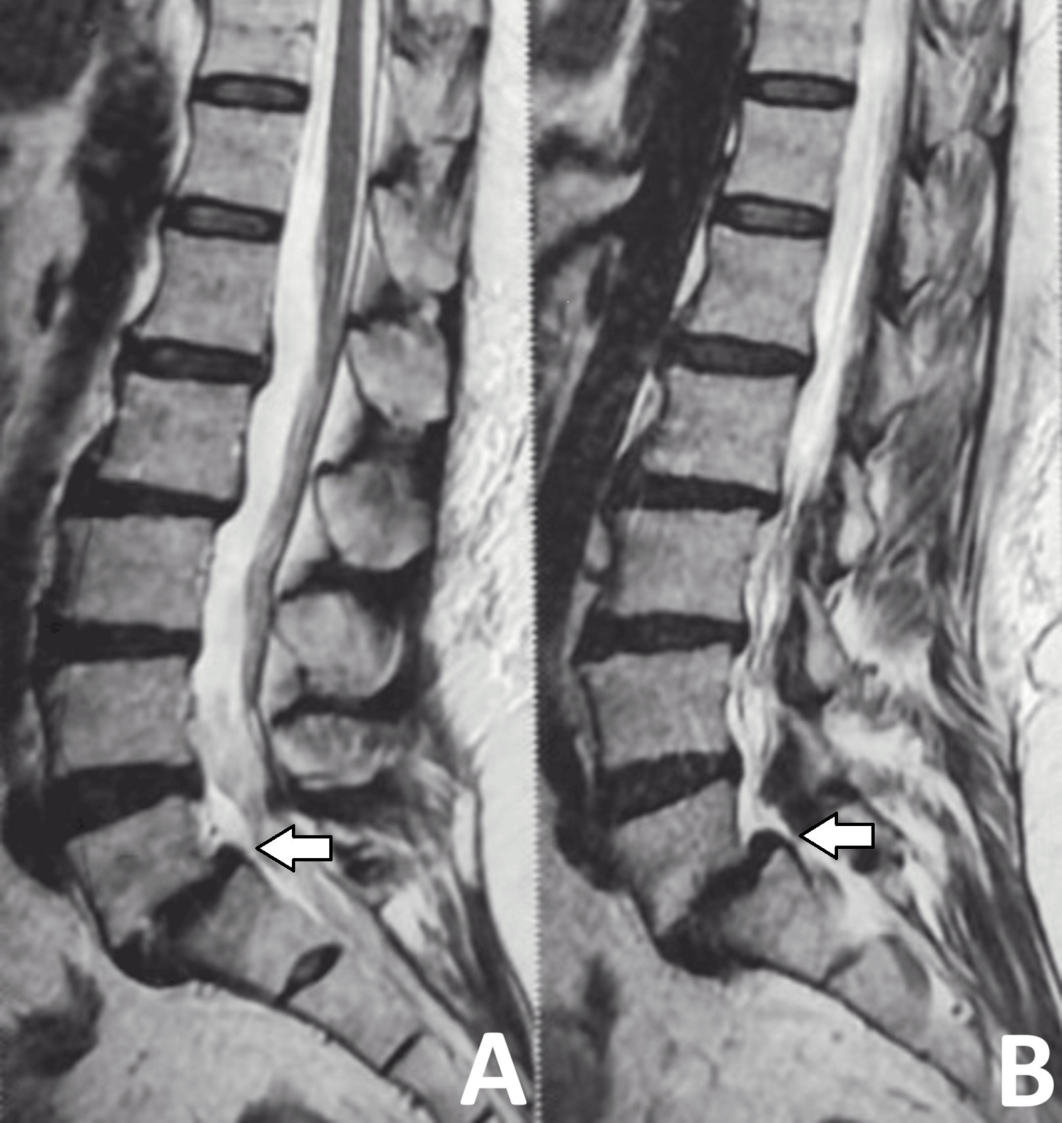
MRI of the lumbosacral spine in sagittal view. T2-weighted MRI of the lumbosacral spine showing L5-S1 spondylolisthesis with a pseudo disc bulge, labeled in two different sagittal sections (A and B).
